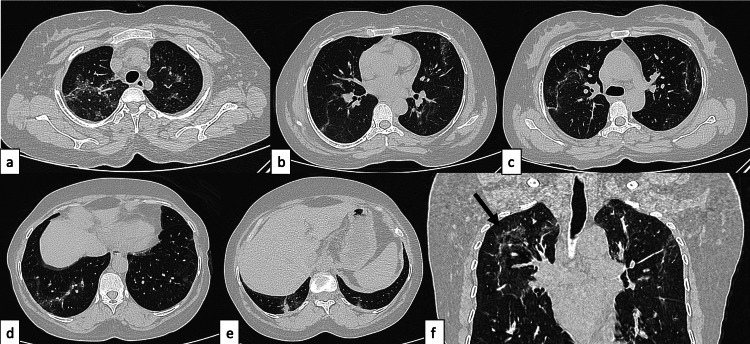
Organising pneumonia A 41-year-old female patient with SLE presented with complaints of shortness of breath, non-productive cough, generalized weakness, and occasional fever of 40-day duration. Axial (a-e) and coronal (f) HRCT chest images in the lung window revealed patchy consolidation and ground-glass opacities in a perilobular pattern, in bilateral lung fields, giving the atoll sign appearance (black arrow). Features were consistent with organising pneumonia. SLE: systemic lupus erythematosus; HRCT: high-resolution CT
